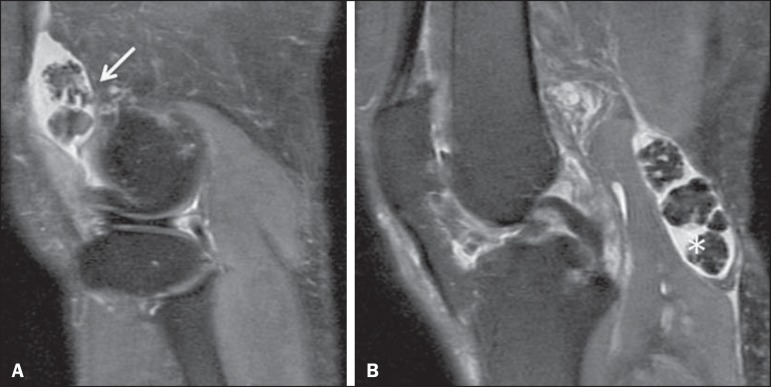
Synovial chondromatosis. Sagittal proton density (PD)-weighted MRI scans
